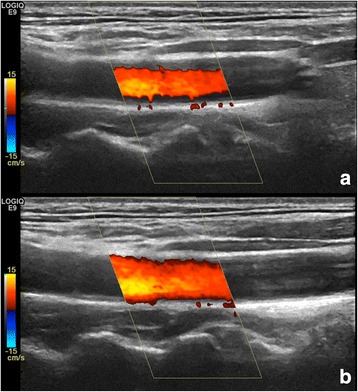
Colour priority/threshold. A low colour priority allows valid GS information to override Doppler information and a high colour priority allows Doppler information to override GS information. In the carotid artery the low colour priority results in poor filling of the vessel lumen (*top*) and should therefore be set high (*bottom*)
